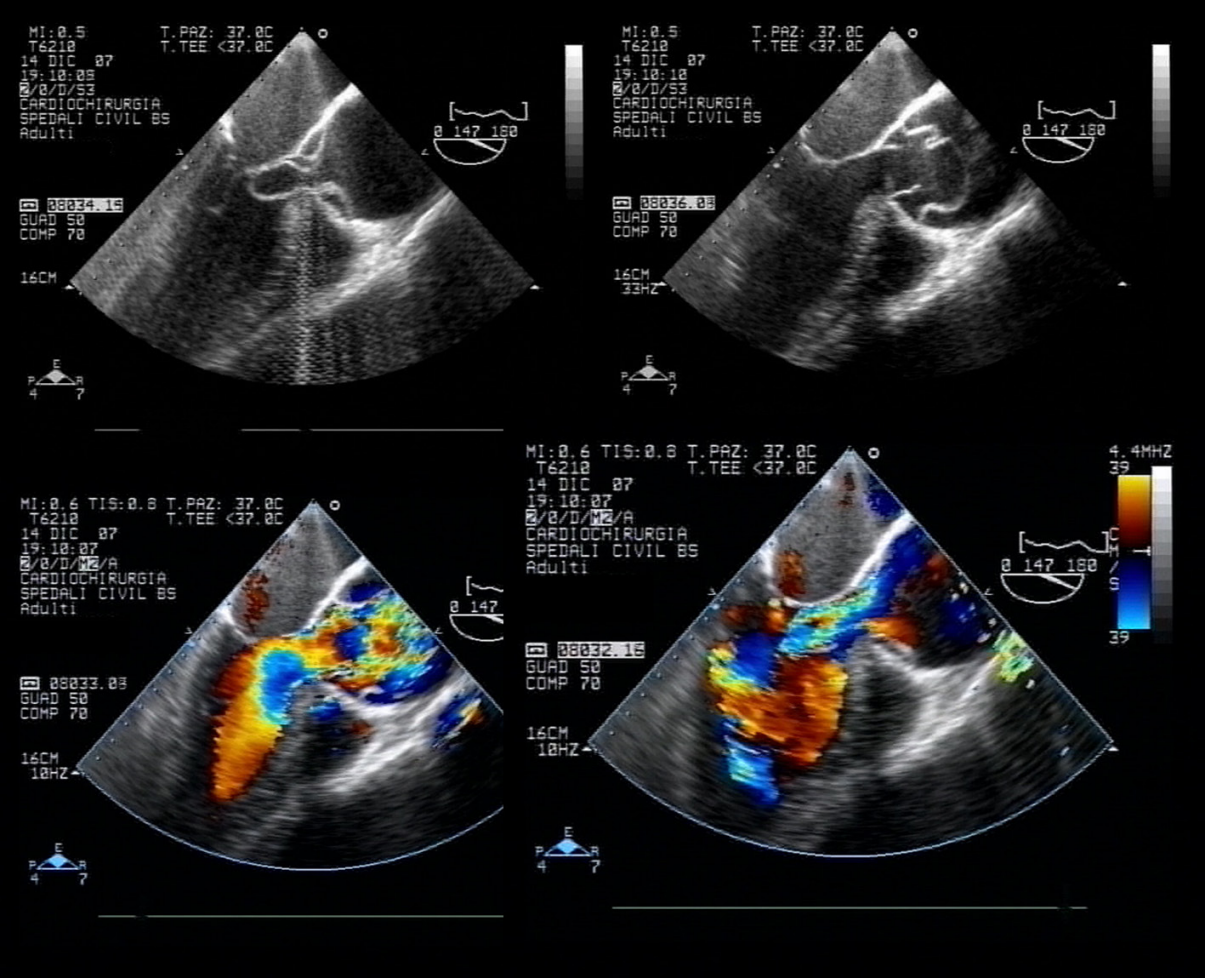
Transesophageal echocardiogram images of aortic dissection.
